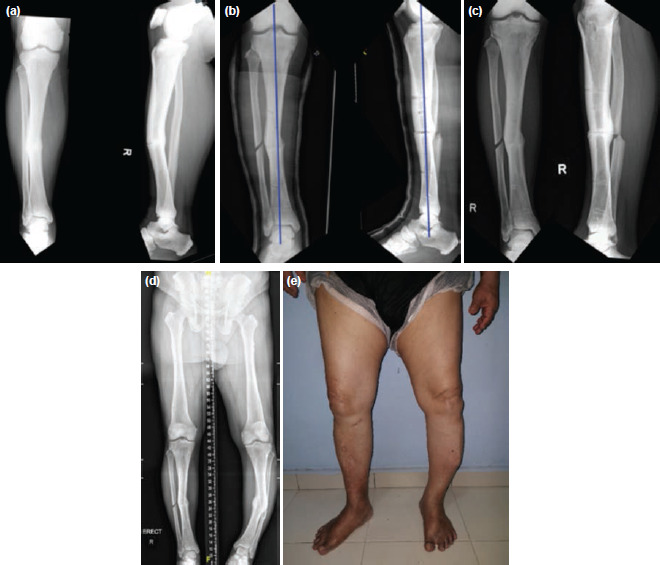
Post-operative radiographs. (a) and (b) Comparison pre-operative (a) and post-operative (b) radiographs. The post-operative radiographs in (b) show complete bony union and correction of the procurvatum and varus deformities are demonstrated by the blue lines indicating the correction of the mechanical axis. (c) AP and lateral radiographs two years after index operation, showing solid union at the non-union site as well as correction of the varus and procurvatum deformities. (d) Long leg film showing the deformity correction at the right lower limb. (e) Clinical photo of the patient’s lower limb with the operated right lower limb demonstrating a comparatively normal mechanical alignment.
